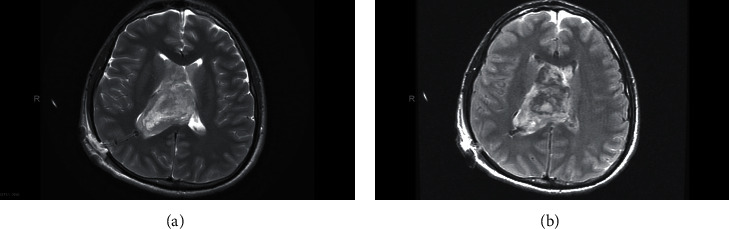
Head MRI axial view. (a) T2. (b) T1.
